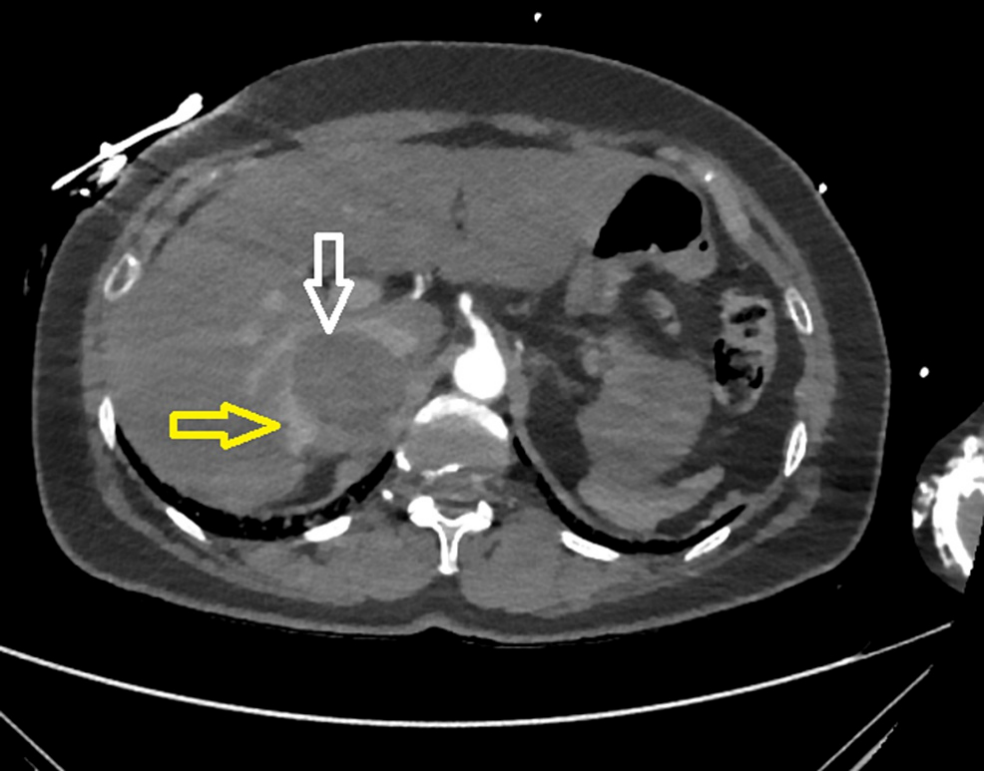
MRI abdomen showing a 5.5 cm mass (white arrow) and an internal bleed (yellow arrow) in the right adrenal gland MRI: magnetic resonance imaging
